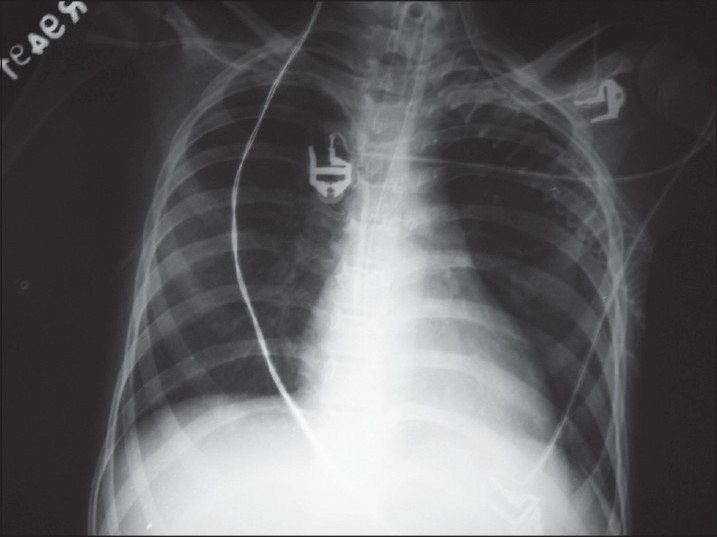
Chest X-ray at time of ICU discharge
